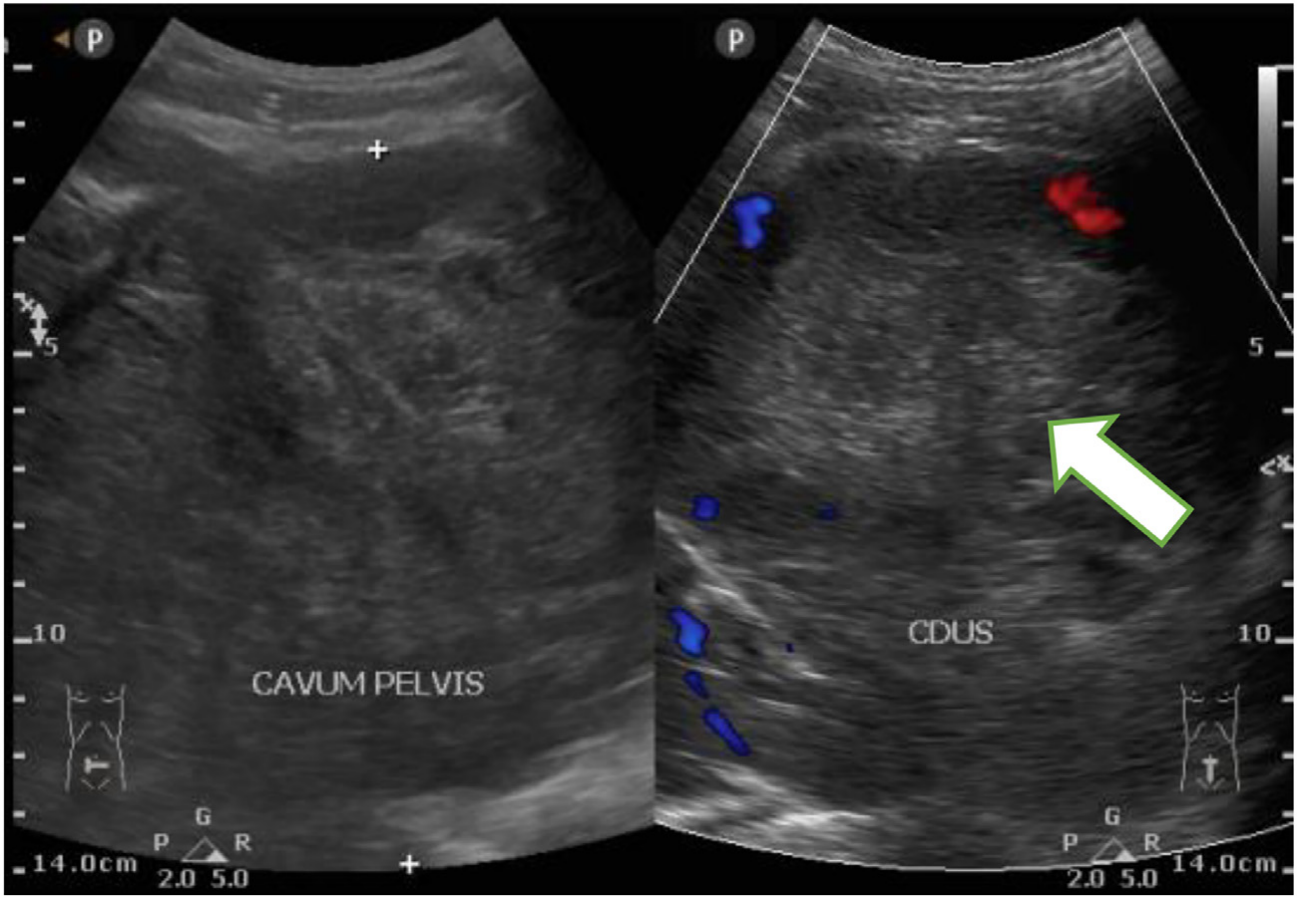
On abdominal ultrasound examination, transverse view showed a large hetero-echoic mass filling the abdominal cavity and pelvic cavity, which on Doppler appeared hypovascular (arrow).
